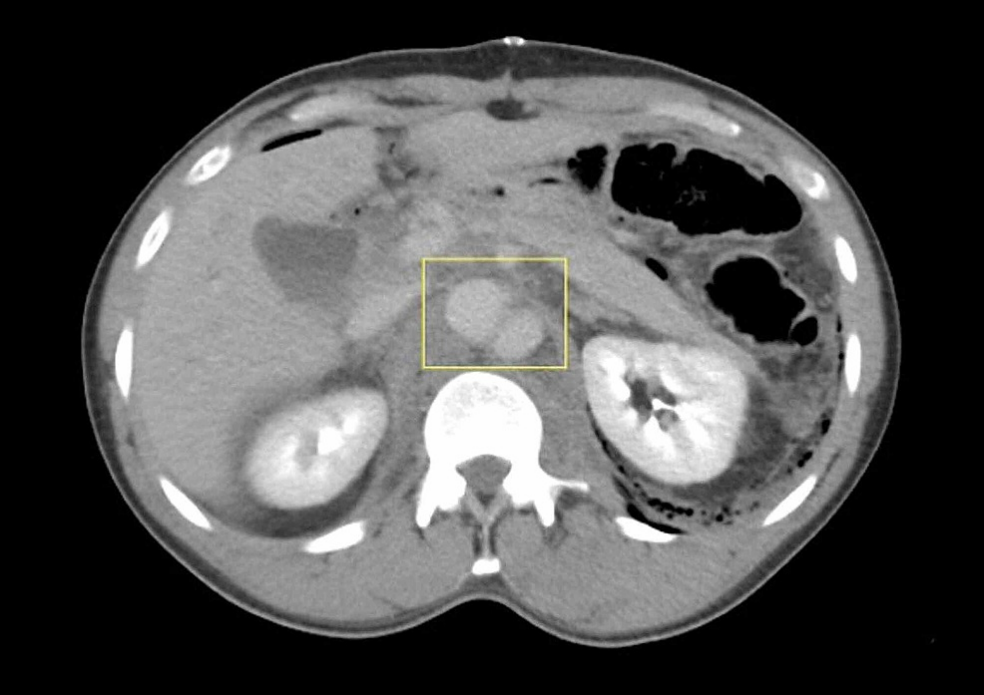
Axial CT showing aortic dissection at the level of the SMA extending to the renal arteries (box) CT: computed tomography; SMA: superior mesenteric artery
